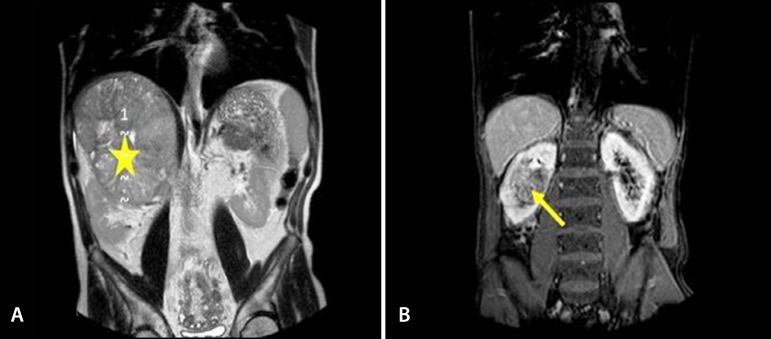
CT image. A=suprarenal tumor (*); B=renal tumor (arrow).
